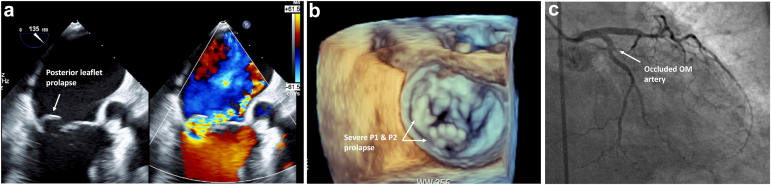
Preprocedural imaging (a) TEE with severe posterior leaflet prolapse and eccentric MR. (b) Three dimensional TEE rendering highlighting P1 and P2 scallop prolapse. (c) Coronary angiography demonstrating occluded OM artery. Abberviations: MR, mitral regurgitation; OM, obtuse marginal; TEE, transesophageal echocardiogram.
